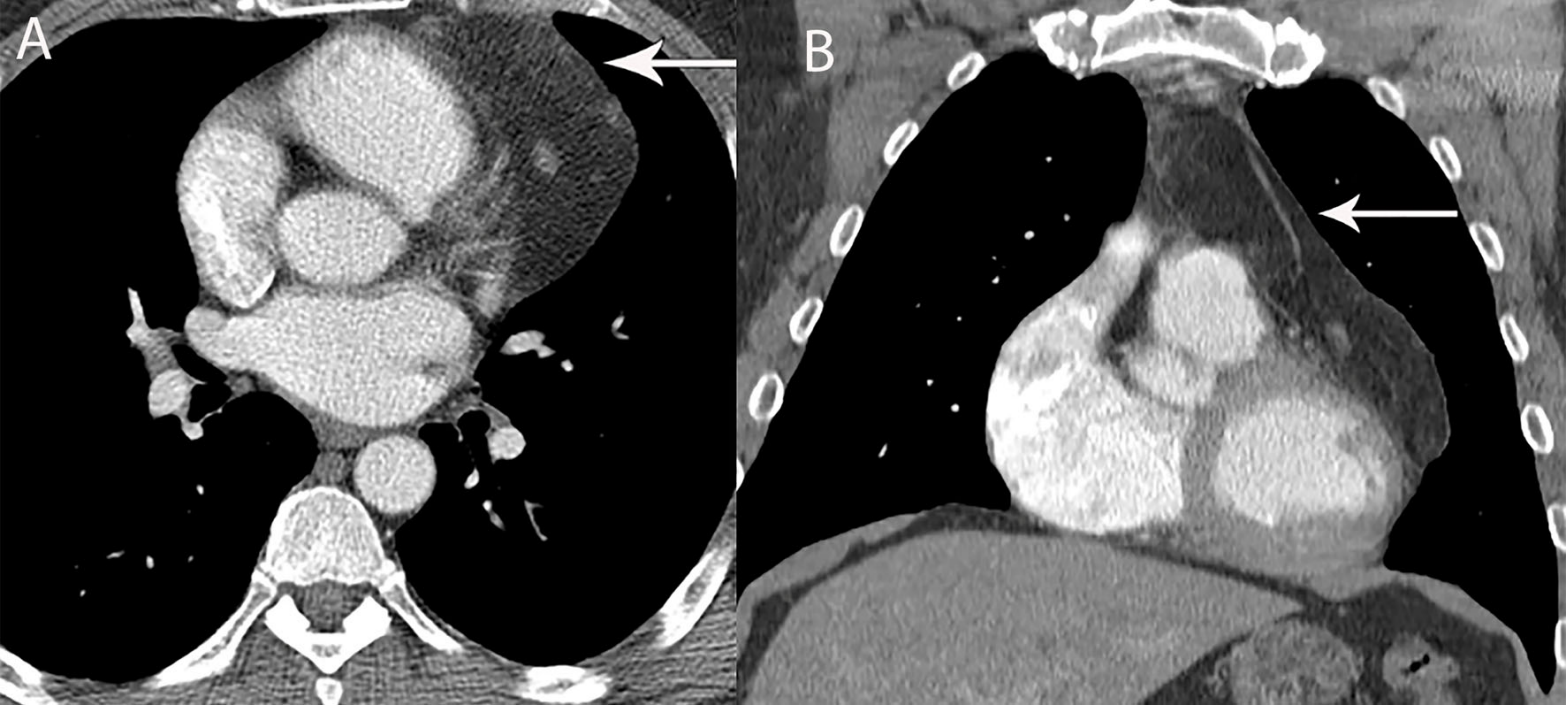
49 year old man with thymolipoma. (A, B) Axial and coronal contrast-enhanced CT shows large left prevascular mediastinal lesion with fat attenuation (arrow).
